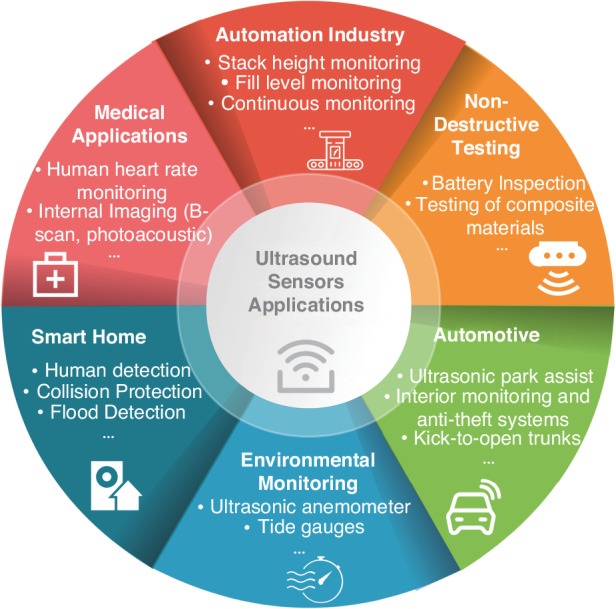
Examples of ultrasound sensor applications
